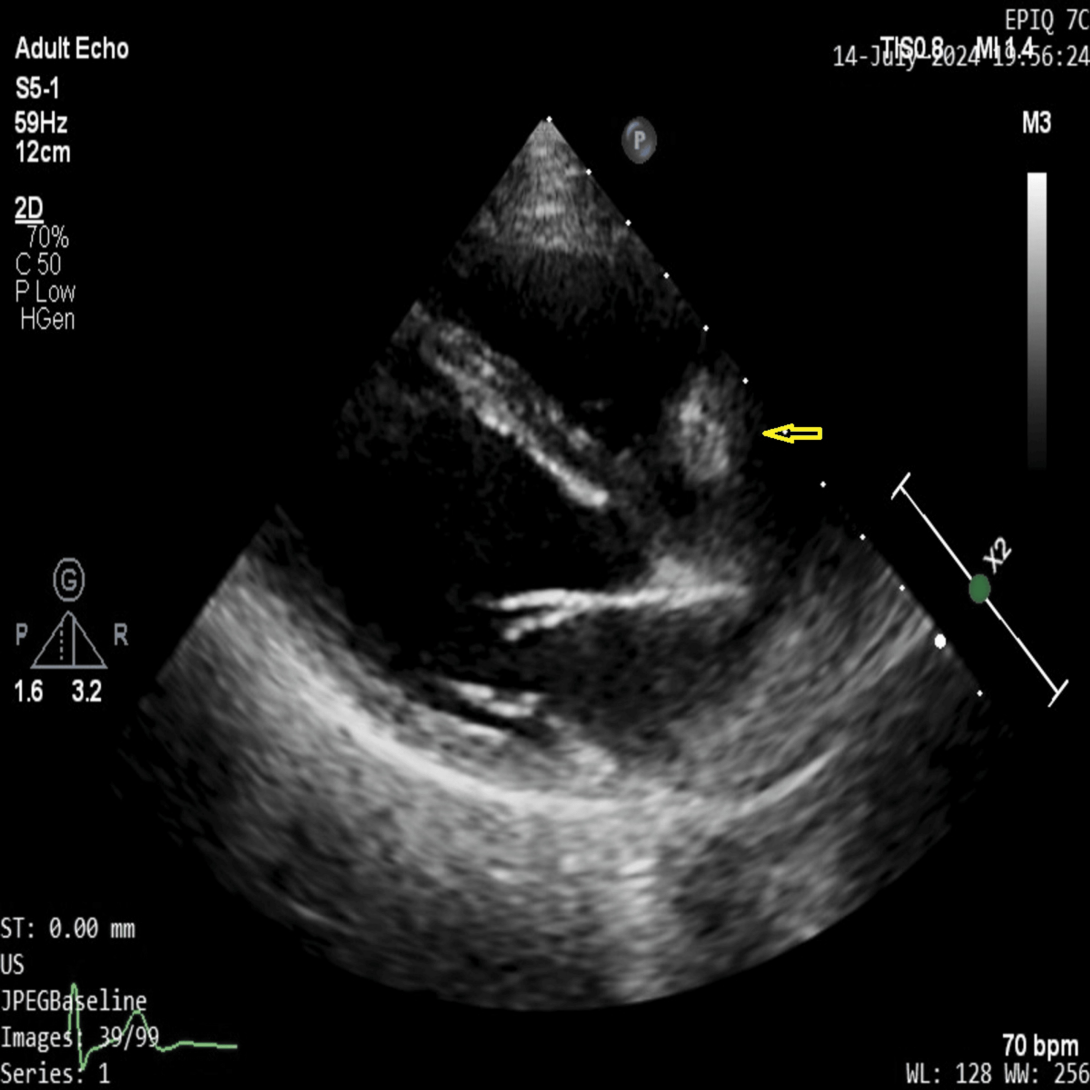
RV inflow view on transthoracic echo showing location of the hyperechoic mass (yellow arrow) in relation to tricuspid valve.
